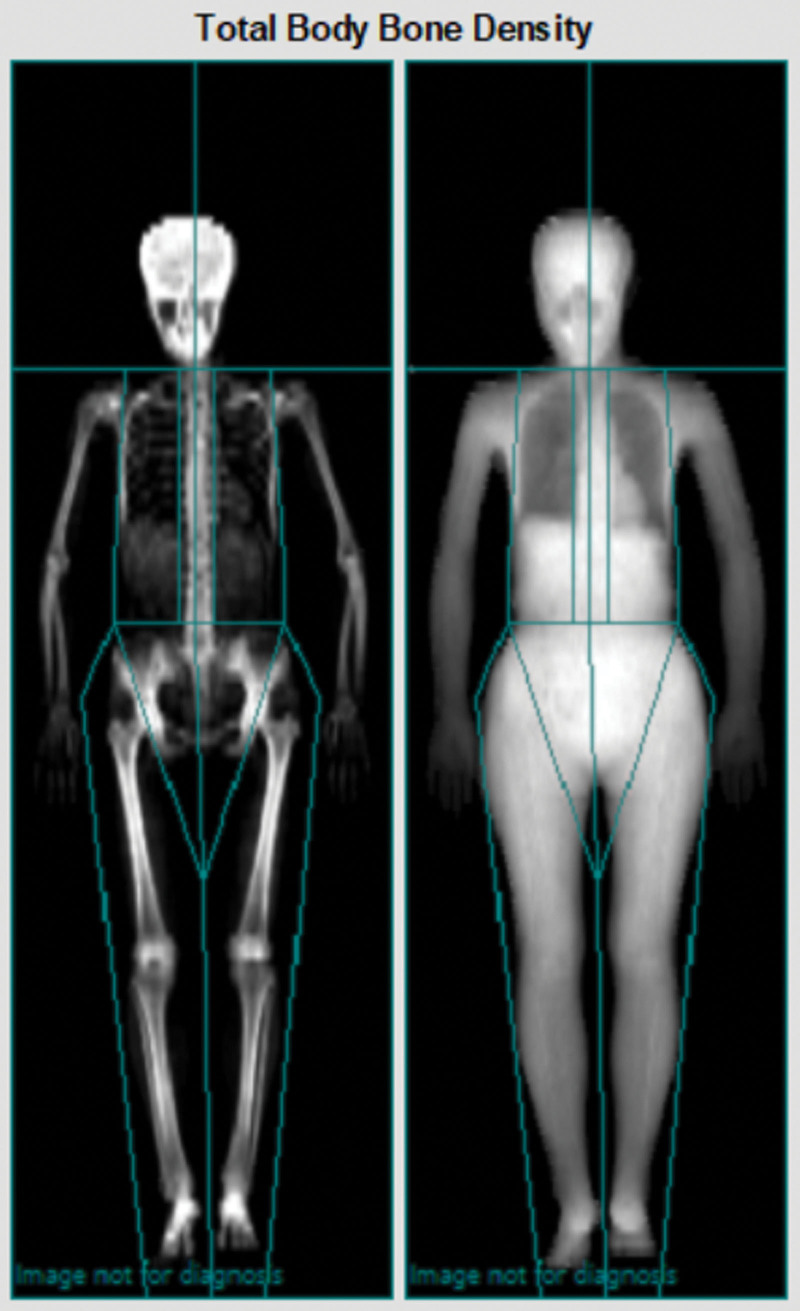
Measuring method of appendicular skeletal muscle mass by dual-energy X-ray absorptiometry.
